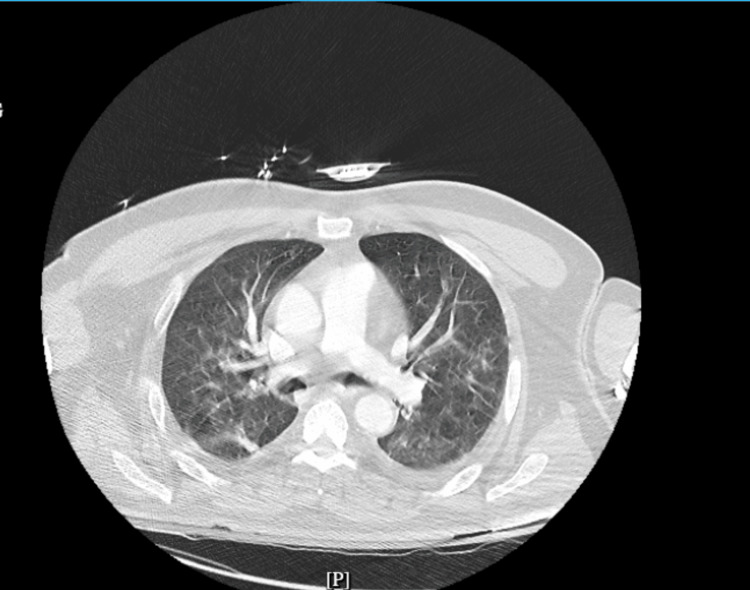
CT angiography of the chest was nondiagnostic for pulmonary embolism but showed small pleural effusions and mild bilateral ground-glass opacities consistent with COVID-19 pneumonitis.
